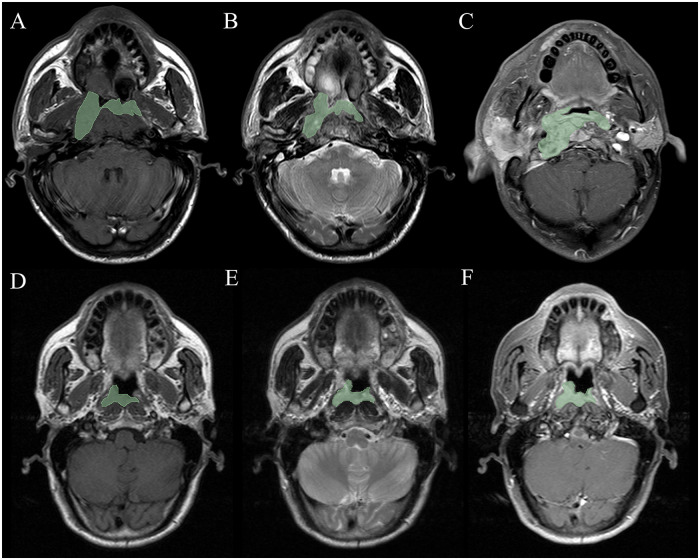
MRI showcase. The figure showcases two patients’ MRI images including T1WI (A,D), T2WI (B,E), and CE-T1WI (C,F) sequences acquired from the opensource dataset (5), which were processed by the manual ROI delineation respectively.
